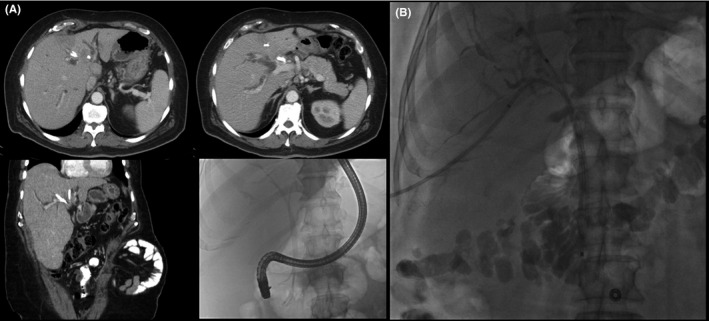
A, Computed tomography and fluoroscopic images showing inappropriate placement of three endoscopic stents in the atrophic left liver (planned resection), without adequate drainage of the future liver remnant (FLR). B, Fluoroscopic image showing super‐selective percutaneous placement of transhepatic catheters into the right anterior and posterior divisions of the FLR
